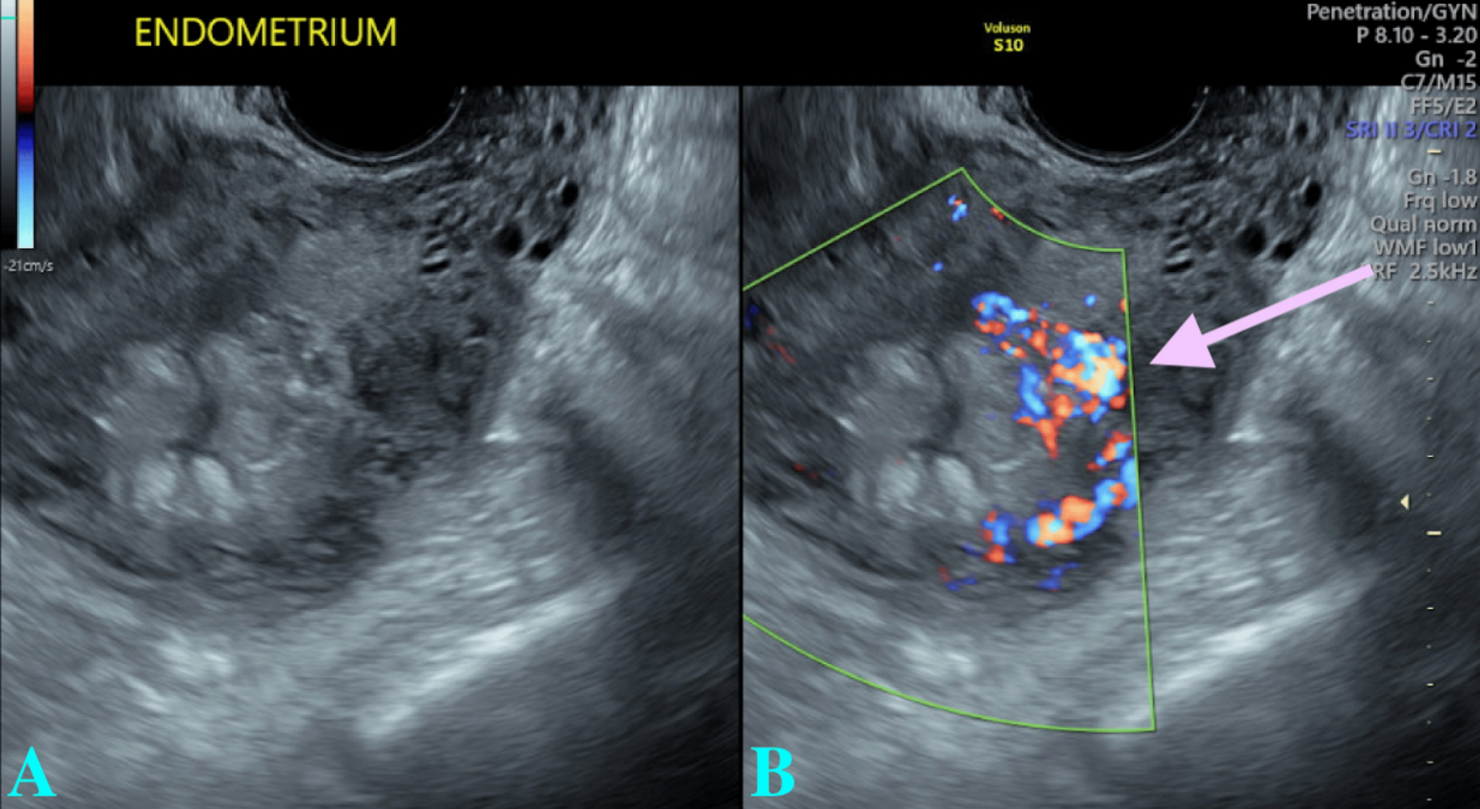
Ultrasound of the endometrium. (A) Endometrium before color Doppler flow. (B) Endometrium after color Doppler flow. The arrow indicates suspected retained products of conception with internal blood flow.
